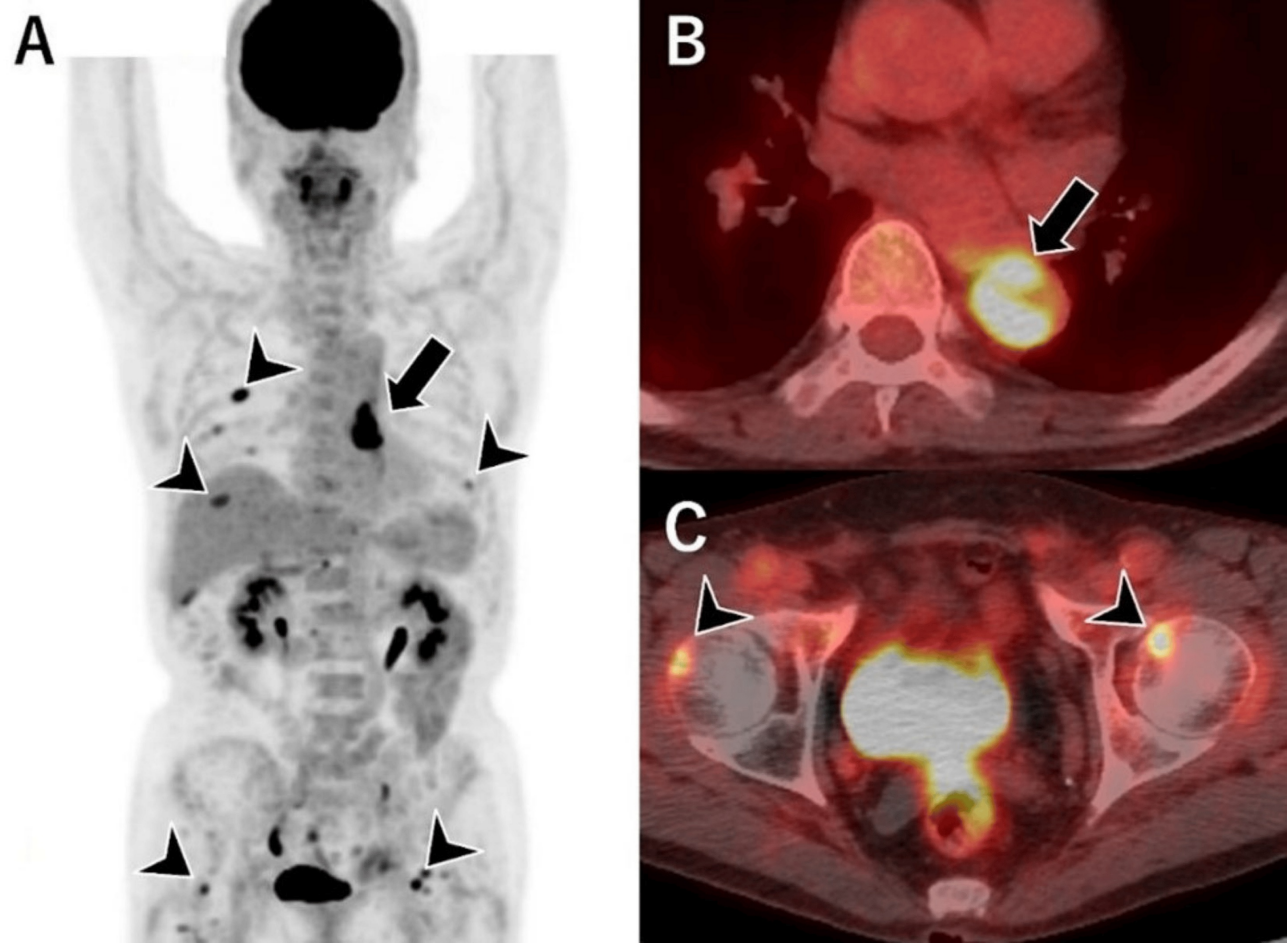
F-18 fluorodeoxyglucose-positron emission tomography (FDG-PET)/CT images. A mass exhibiting abnormal accumulation, with a maximum standardized uptake value of 10.8, is identified in the descending aorta (A, B: arrows), along with additional metastatic lesions observed in the ribs and femoral heads (A, C: arrowheads).
